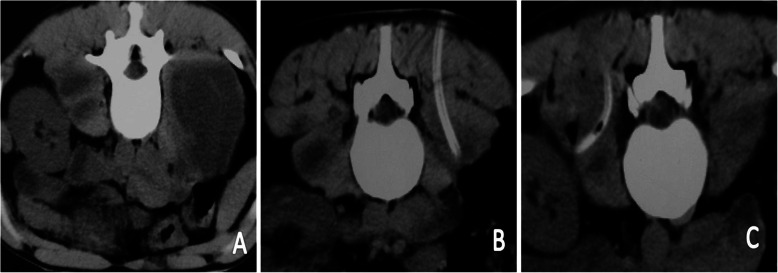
(a) The bilateral psoas abscess cavities were observed in the process of percutaneous puncture. (b) and (c) The abscess drainage tubes were placed in the middle of the abscess cavities under CT-guidance
